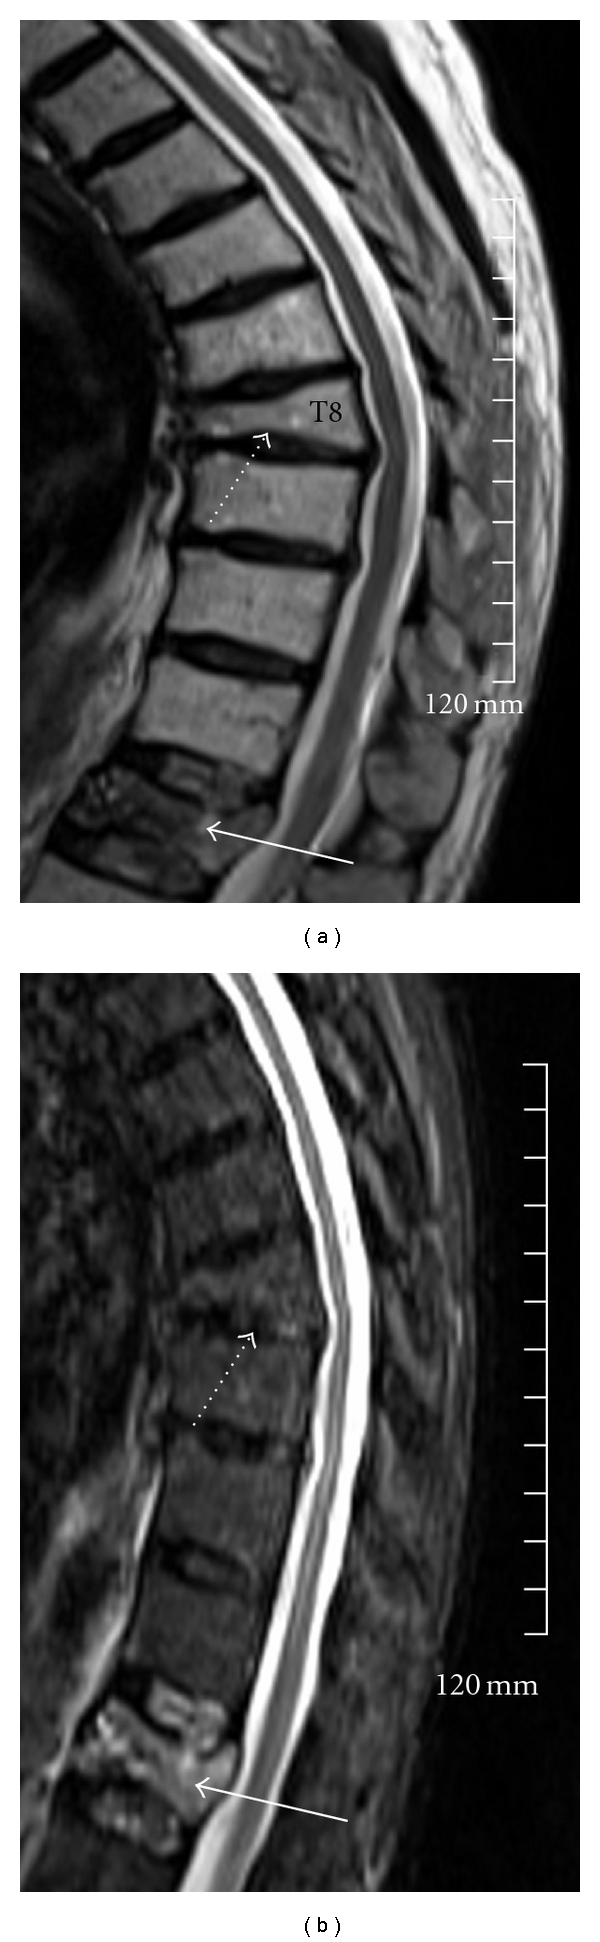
Sagittal TSE T2-weighted (a) and sagittal STIR (b) of the thoracolumbar spine in a 56-year-old man referred for PV for severe back pain following a fall. VCFs are noted at T8 (white dashed arrow) and T12 (continuous white arrow). There is prominent STIR hyperintensity at T12 without significant marrow edema at T8. The patient was symptomatic over T12 on palpation. This level was cemented with complete resolution of symptoms.
